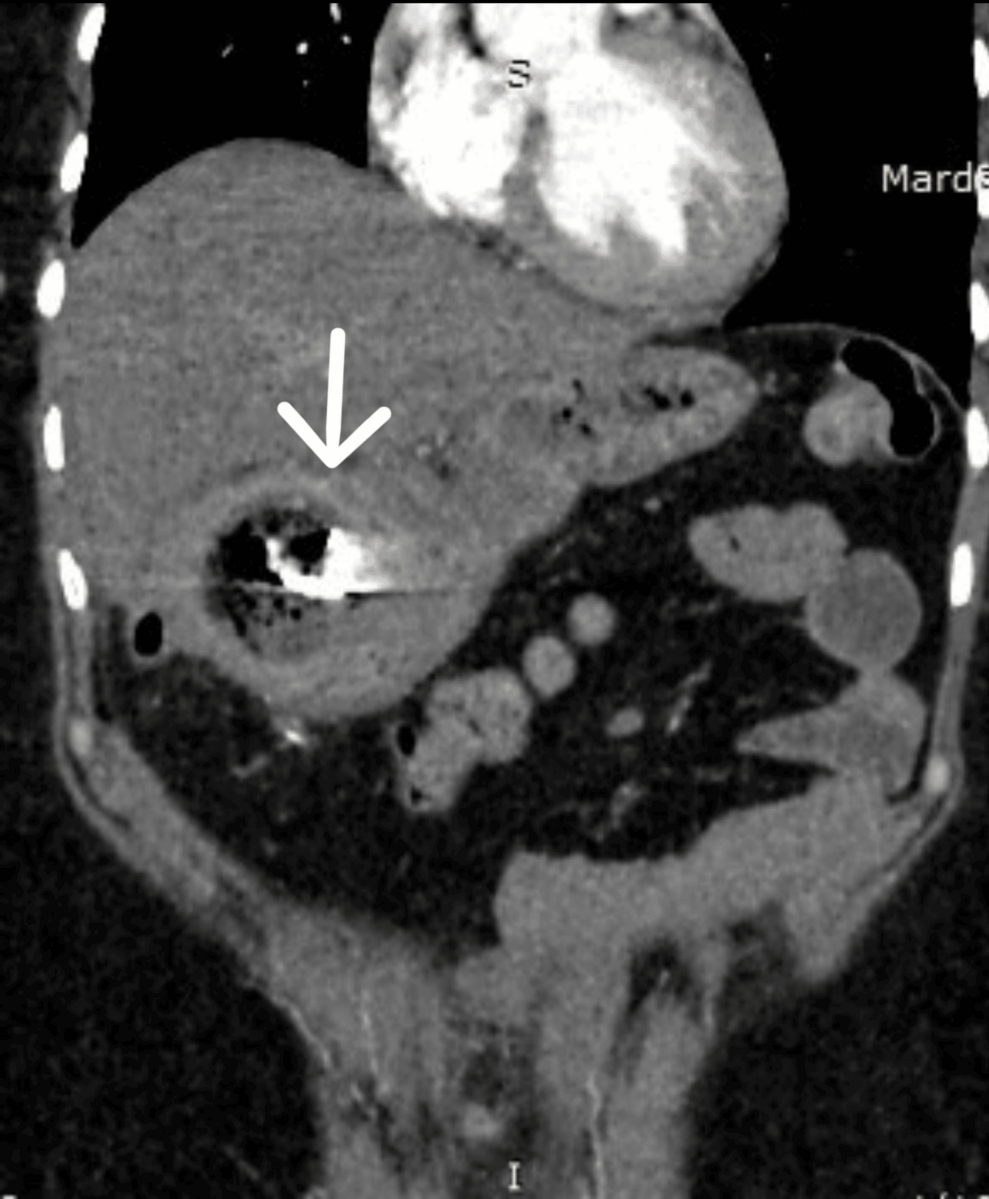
CT (coronal view) of the abdomen and pelvis showing the retained surgical pack (arrow)
